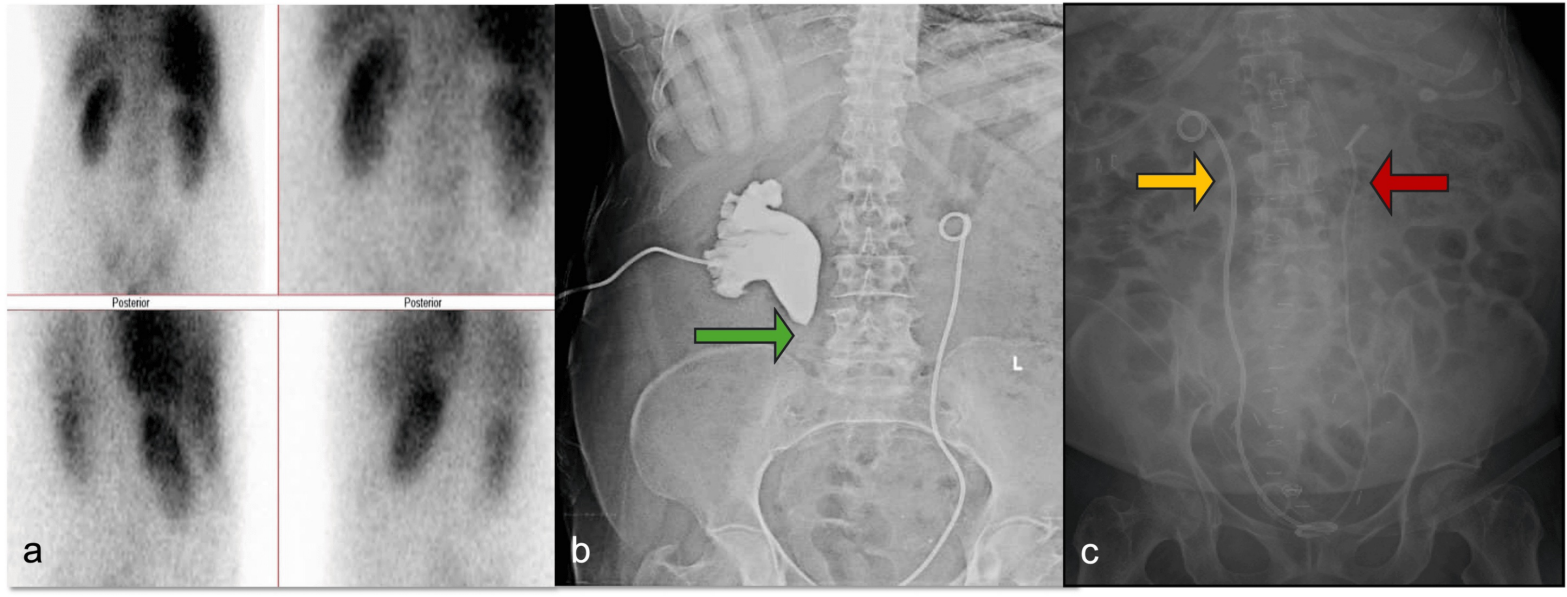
Nuclear imaging (a) and X-ray images of the kidney, ureter, and bladder (KUB) (b, c) (a) Bilateral renal scarring with reduced renal function. (b) Nephrostogram showing complete ureteral occlusion at the pelvi-ureteric junction level (green arrow). (c) Kidney, ureter, and bladder (KUB) X-ray showing 8 Fr stent on the right side (yellow arrow) and 6 Fr stent on the left side (red arrow).
